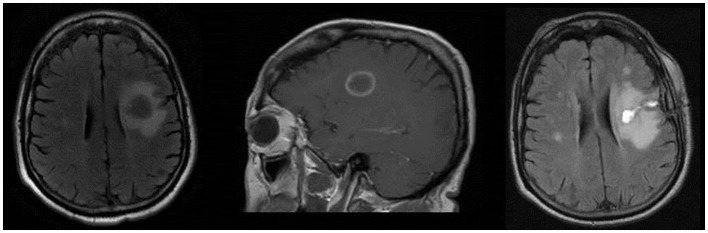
Left image: axial T2 FLAIR showing 2 cm × 2.3 cm left frontal mass with substantial edema. Middle image: sagittal T1 post-contrast image showing contrast enhancement in a “ring-like” pattern. Right image: axial T2 FLAIR showing residual changes consistent with partial resection of the previous ring-enhancing left frontal mass.
